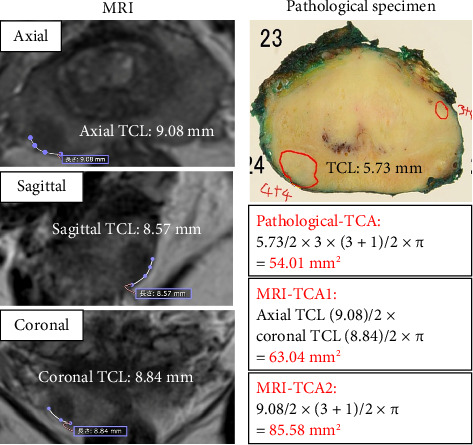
An example of MRI-TCL, MRI-TCA1, MRI-TCA2, pathological-TCL and pathological-TCA.
